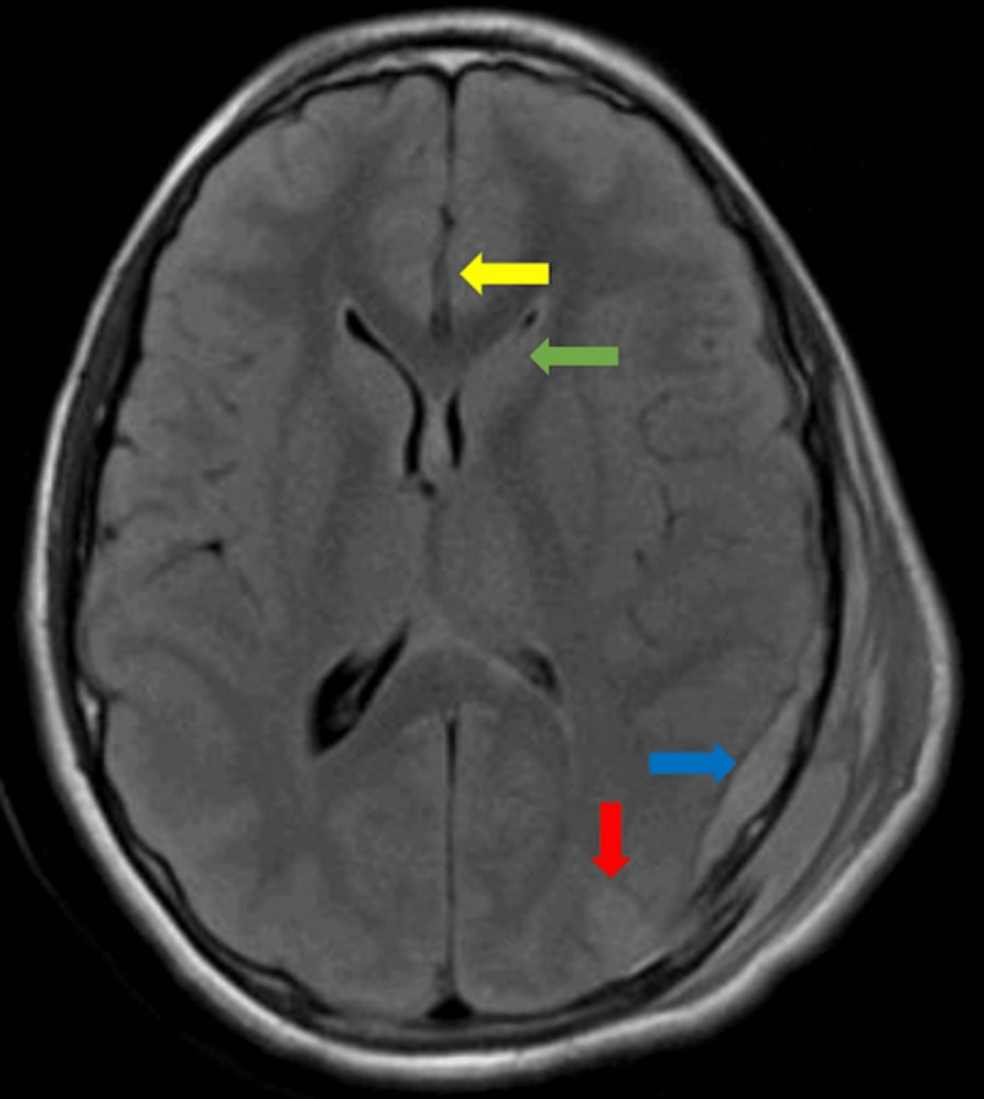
Magnetic resonance imaging of the brain with subdural hematoma (blue arrow), parenchymal hemorrhagic contusion (red arrow), mass effect on the frontal horn of the left lateral ventricle (green arrow), and rightward midline shift (yellow arrow).
